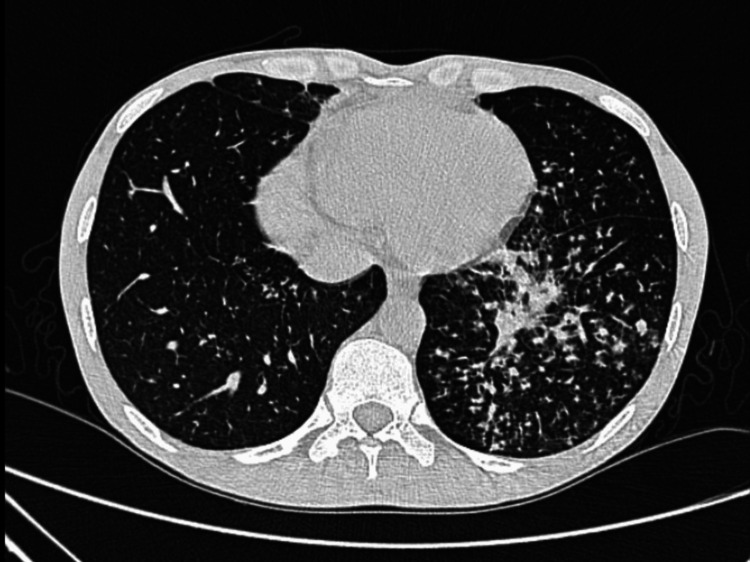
HRCT image showing ill-defined centrilobular nodules with a tree-in-bud appearance, some coalescing to form a consolidative patch, suggestive of active pulmonary tuberculosis HRCT, high-resolution CT
